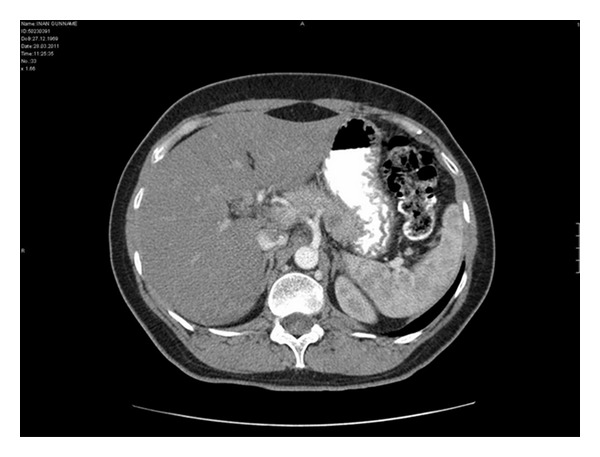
Abdominal CT shows a 4 × 2 cm-sized cystic lesion in mid portion of pancreas.
